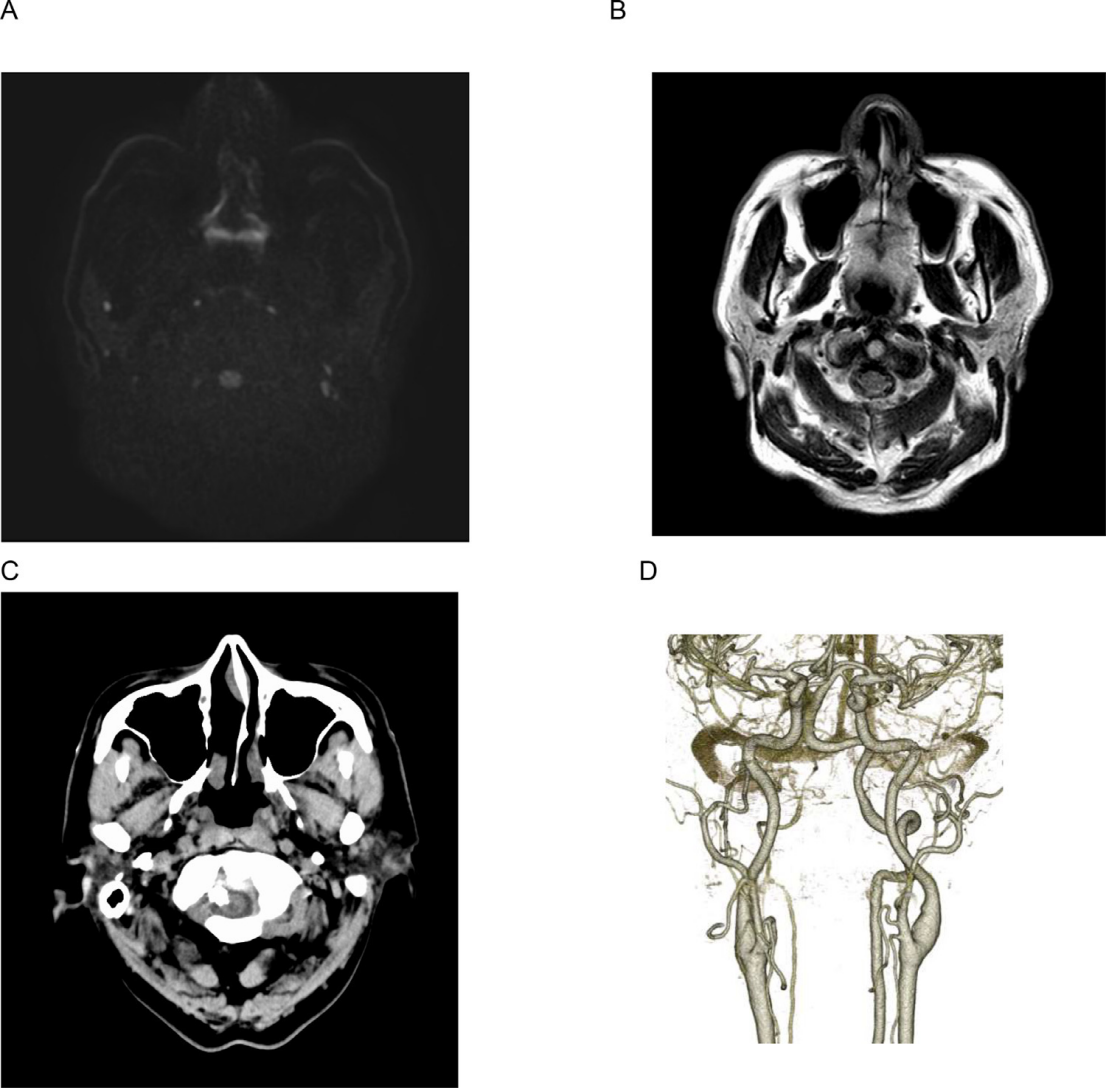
(A) A postprocedural MRI-DWI shows no evidence of spinal infarction. DWI: diffusion weighted image. (B) A postprocedural MRI-FLAIR shows no evidence of high intensity lesions. FLAIR: fluid attenuated inversion recovery. (C) A postprocedural CT image shows no evidence of hemorrhage and the presence of NBCA casts along the spinal nerve root. (D) Postoperative CT angiography image confirmed no evidence of abnormal blood vessels around the posterior cranial fossa.
